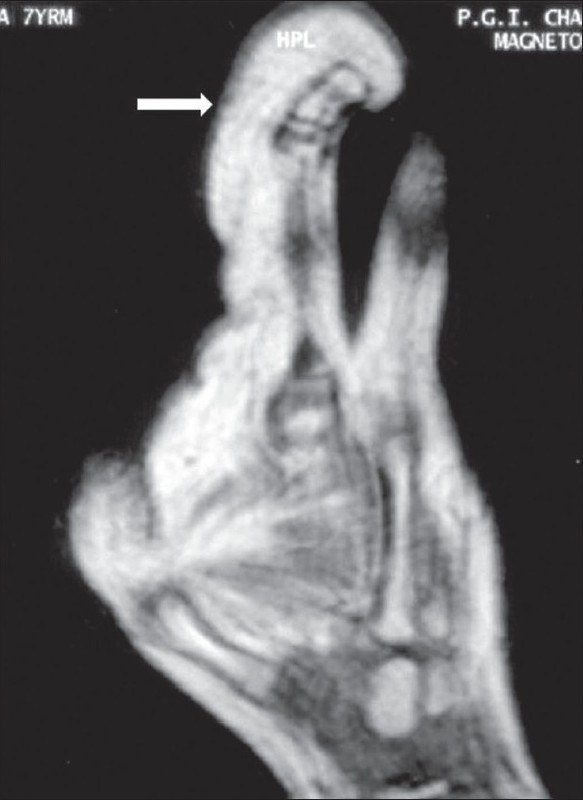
Case 1: Sagittal T1W MRI image reveals proliferation of fatty tissue (arrows) on the volar aspect of the index finger with signal intensity similar to that of subcutaneous fat; there is dorsal angulation of the affected digit
